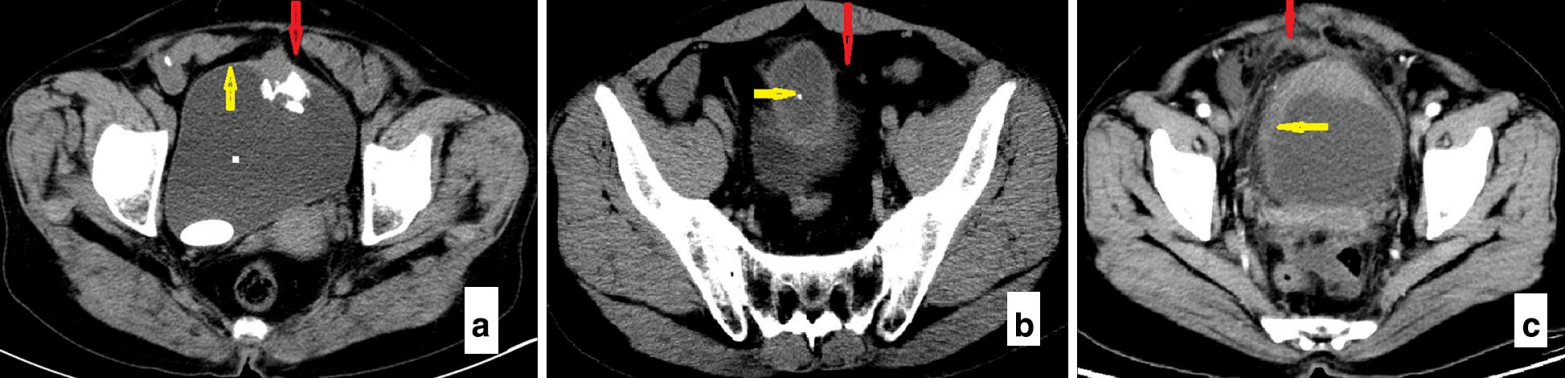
Computed tomography (CT) manifestations of a urachal carcinoma and a benign urachal mass. a On an axial view of a urachal carcinoma, the *red arrow* indicates the Retzius space without fat infiltration, and the *yellow arrow* indicates a lack of thickening of the surrounding bladder wall. b On an axial view of a urachal carcinoma, the *red arrow* indicates the Retzius space without fat infiltration, and the *yellow arrow* indicates calcification in central lesions. c On an axial view of a benign urachal mass, the *red arrow* indicates fatty infiltration of the Retzius space, characterized by enhancements of some patches or strips and vague indications of fat surrounding the lesions, and the *yellow arrow* indicates diffuse thickening of the bladder wall not only around the lesions but also across the whole bladder dome and two side walls, with the peripheral wall gradually thinning to normal
